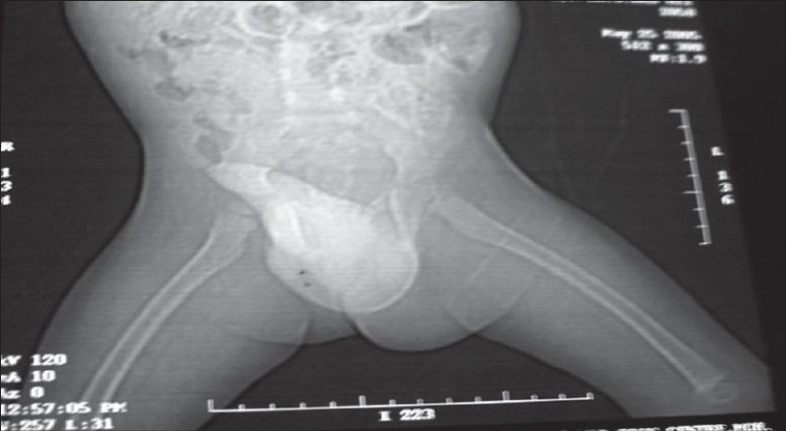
CT reconstruction showing the mass
